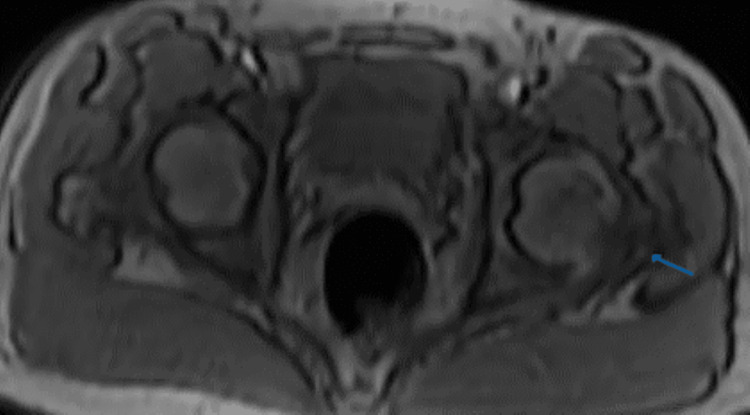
Both hip MRI findings: right sacroiliitis with trace fluid around the iliacus and multifidus muscles. Minimal left hip joint effusion.
